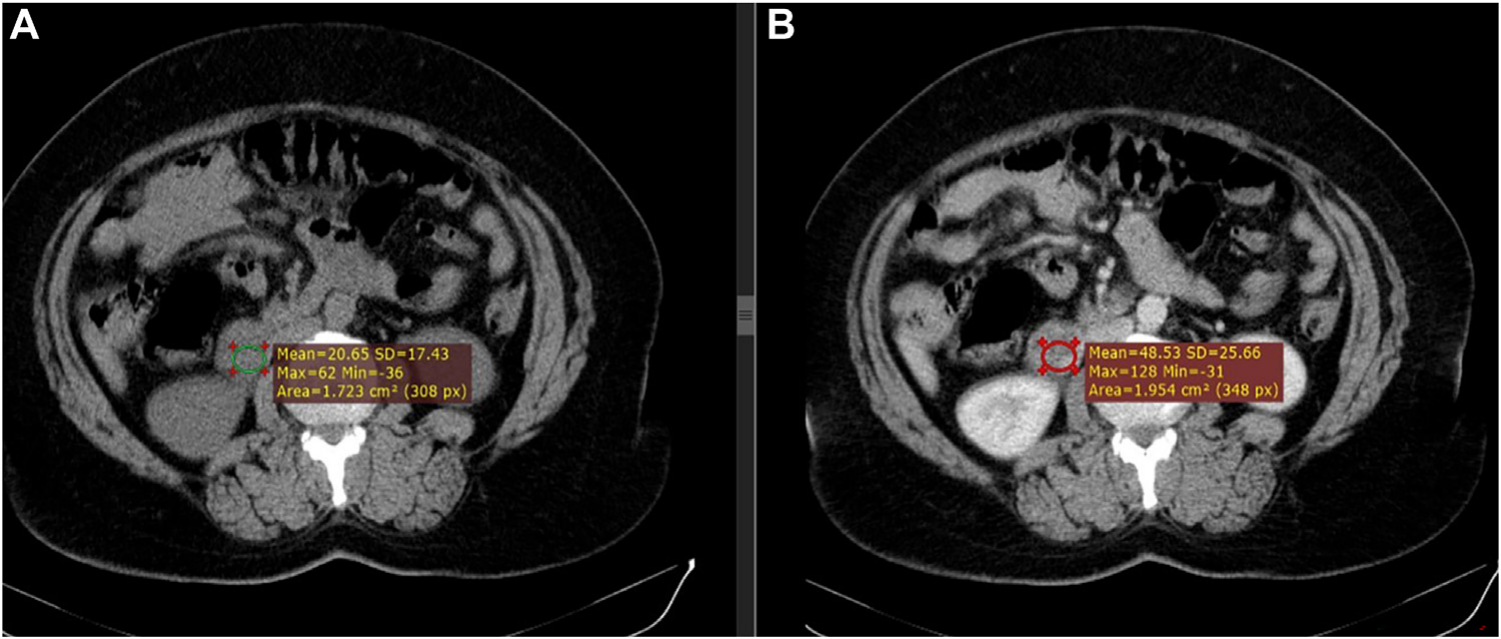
Contrast-enhanced CT images of the abdomen. Portal venous (green circle) and Excretory phases show a heterogeneously enhanced mass (red circle). Structures surrounding the lesions are: medially the inferior vena cava; posteriorly right gonadal vein and right ureter, anteromedially duodenum, anterolaterally mesenteric fat, laterally right kidney and ascending colon. The density of the lesion in the native scan is 20 HU, and in the postcontrast scan is 48HU.
